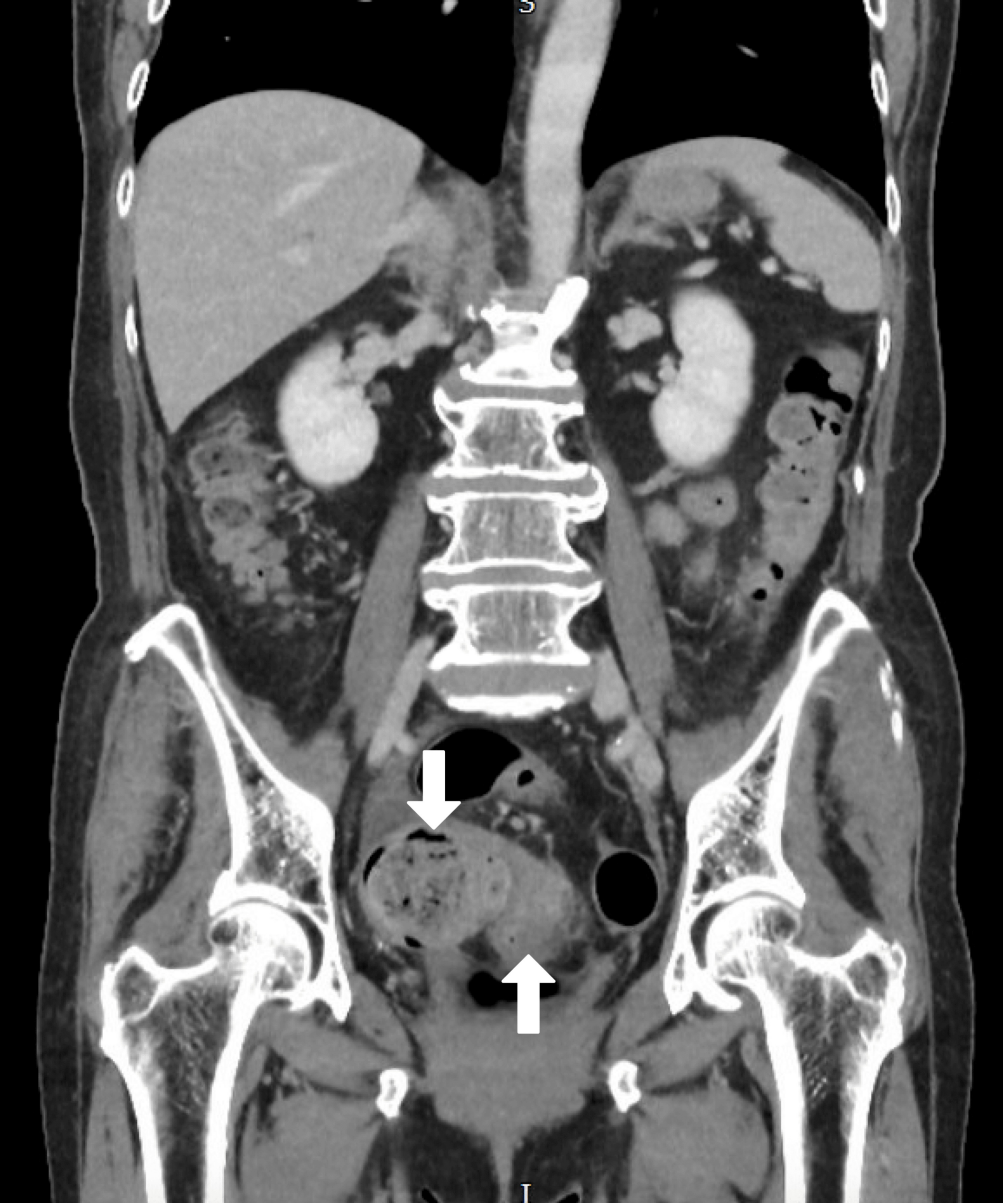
CT Abdomen & Pelvis (Coronal View)
